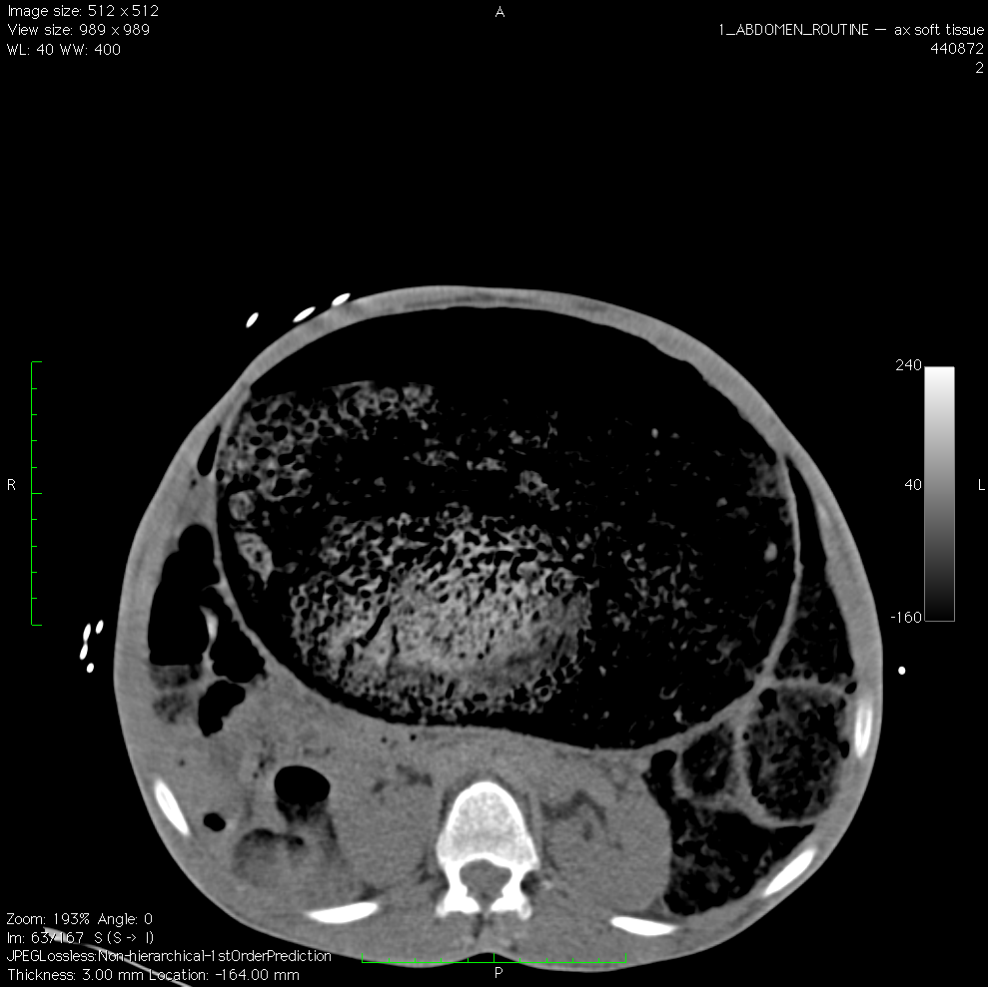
Axial CT image of the pseudo-obstruction and severely dilated colon.
